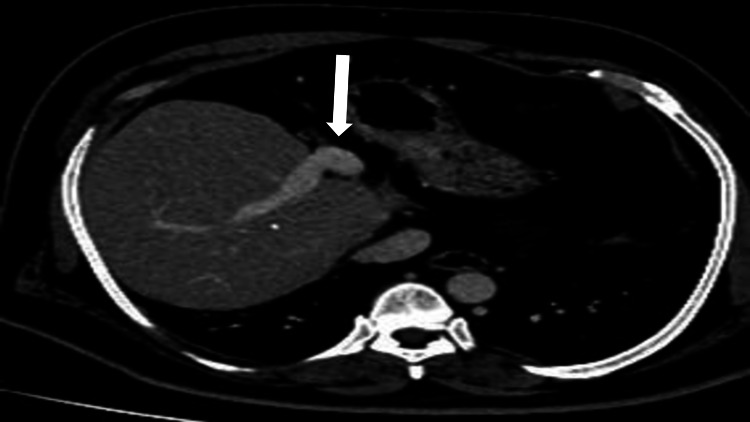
CT postcontrast axial view shows the main portal vein and its right branch. The left portal vein is not visualized.
